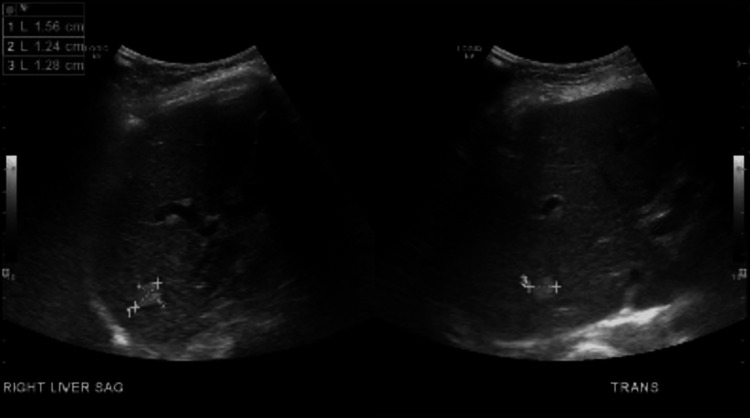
The ultrasonography of the liver showed a 1.6 cm hepatic lesion.
